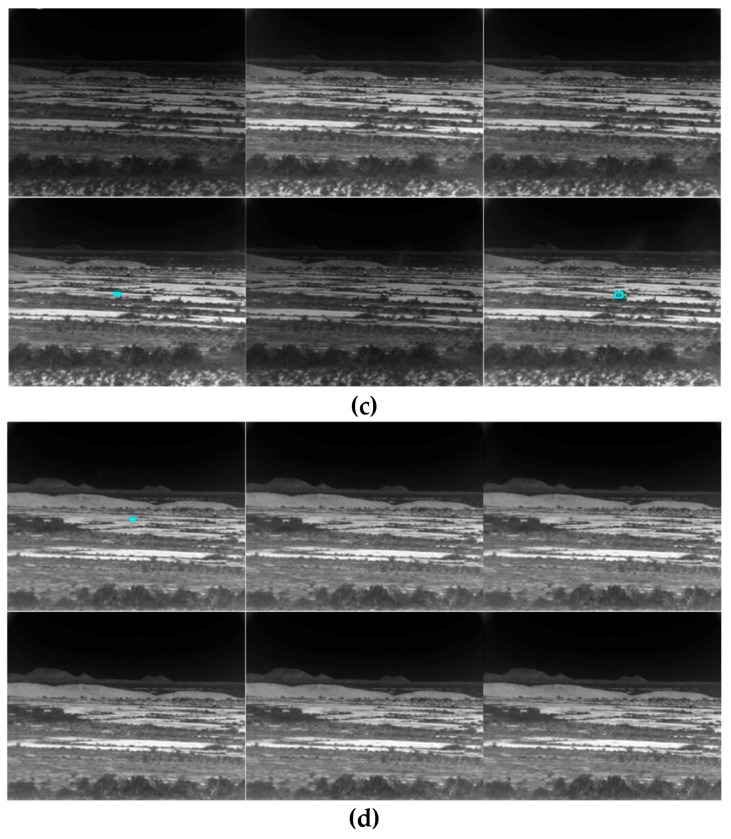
Tracking results for frames 1, 60, 119, 178, 237, and 296 for the PCE full (MWIR daytime) case. The vehicle is SUV. Only some frames have detections. (a) 1000 m; (b) 2000 m; (c) 2500 m; and (d) 3500 m.
